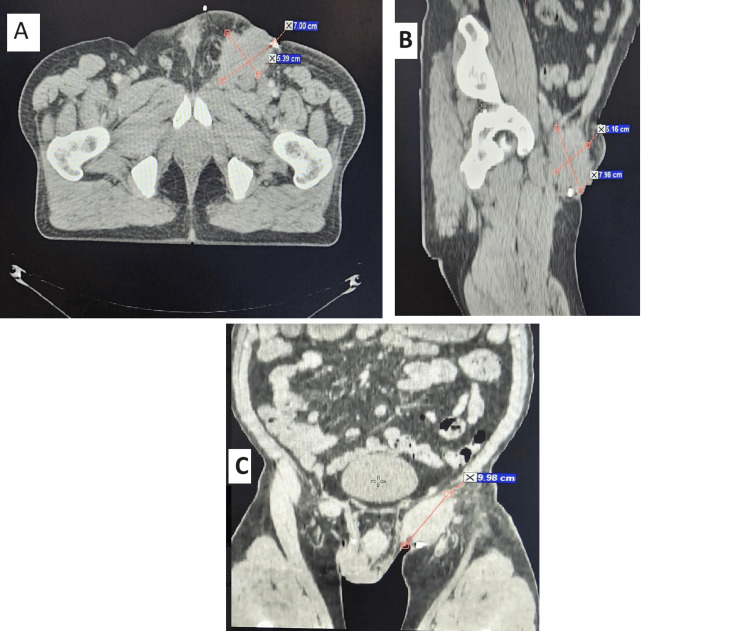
Axial (A), sagittal (B), and frontal (C) CT scan identified a left inguinal tumoral process invading the adjacent soft tissues and infiltrating the left spermatic cord with right inguinal adenopathy and left external iliac adenopathy.
